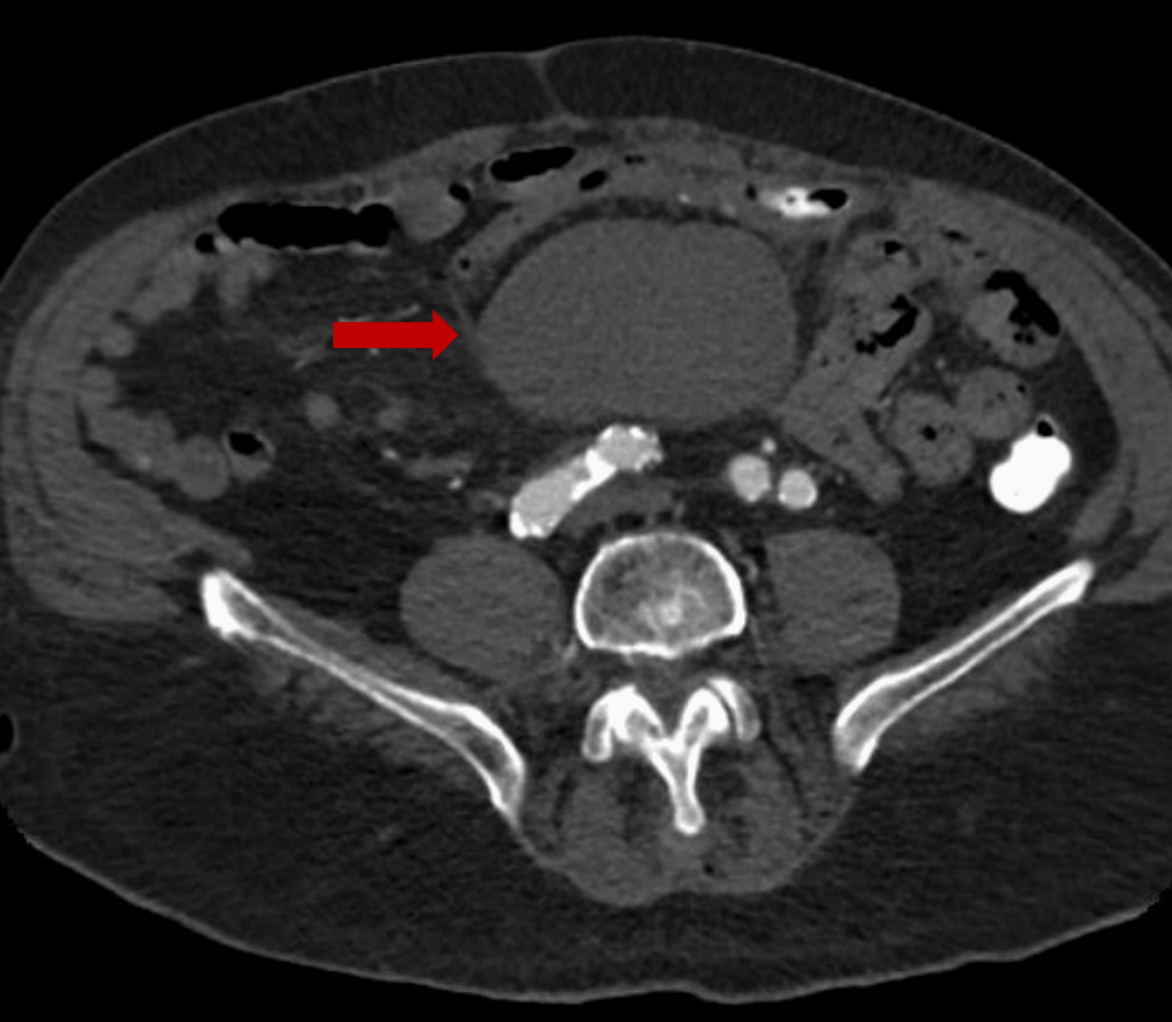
Abdominal Computed Tomography Scan of the Mesenteric Hematoma Red arrow: mesenteric hematoma
